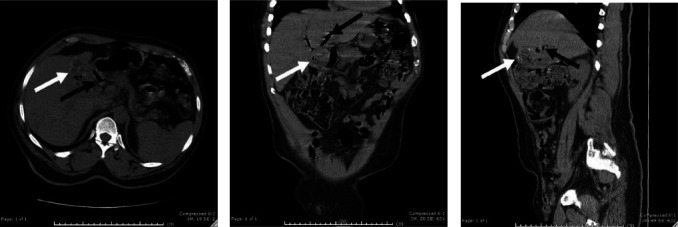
Computerized tomography (CT) images of the abdomen and pelvis without contrast: (A) axial image, (B) coronal image, and (C) sagittal image. White arrow denotes the gallbladder with emphysematous cholecystitis. Black arrow denotes pneumobiila.
